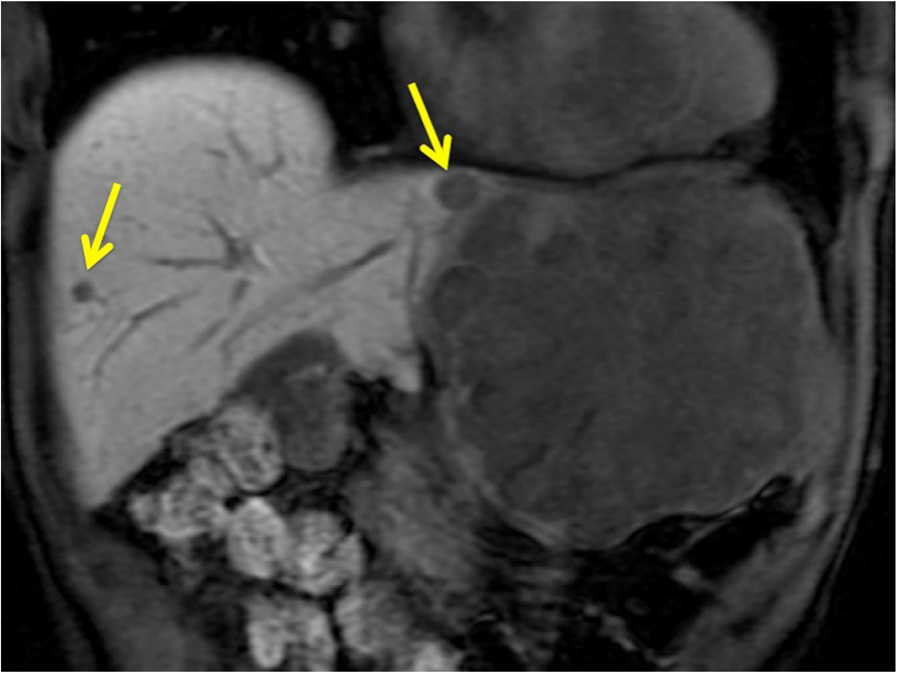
MRI demonstrated a large tumor and multiple intrahepatic metastases (arrow)
